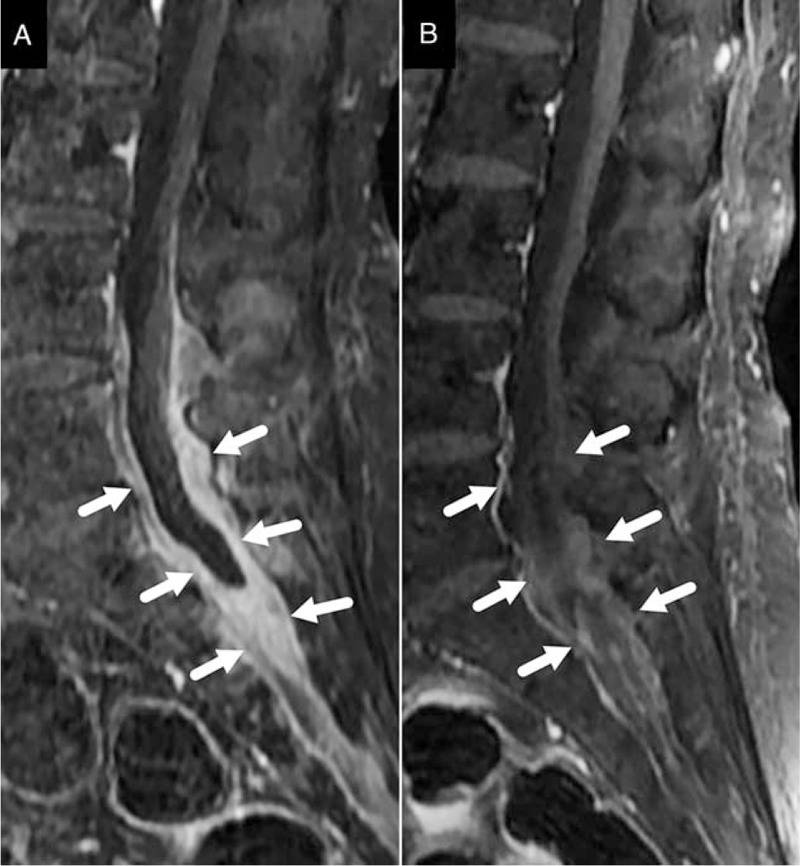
Case 2. Sagittal Gd-enhanced T1-weighted magnetic resonance images. (A) Images taken in November 2014, demonstrating diffusely thickening of dura mater at L4 and S3 level with marked enhancement (arrow). (B) Images taken in February 2015, showing that the thickened dura mater significantly improved after immunosuppressive therapy (arrow).
